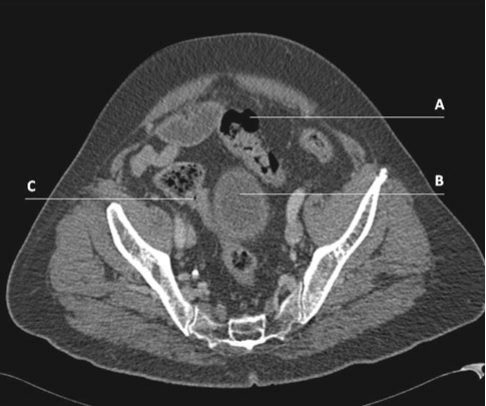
Preoperative CT image without evident signs of free fluid or generalized peritonitis of a patient who appeared to have Hinchey 3 perforated diverticulitis during surgery. *A* free air; *B* bladder; *C* colonic diverticulum
